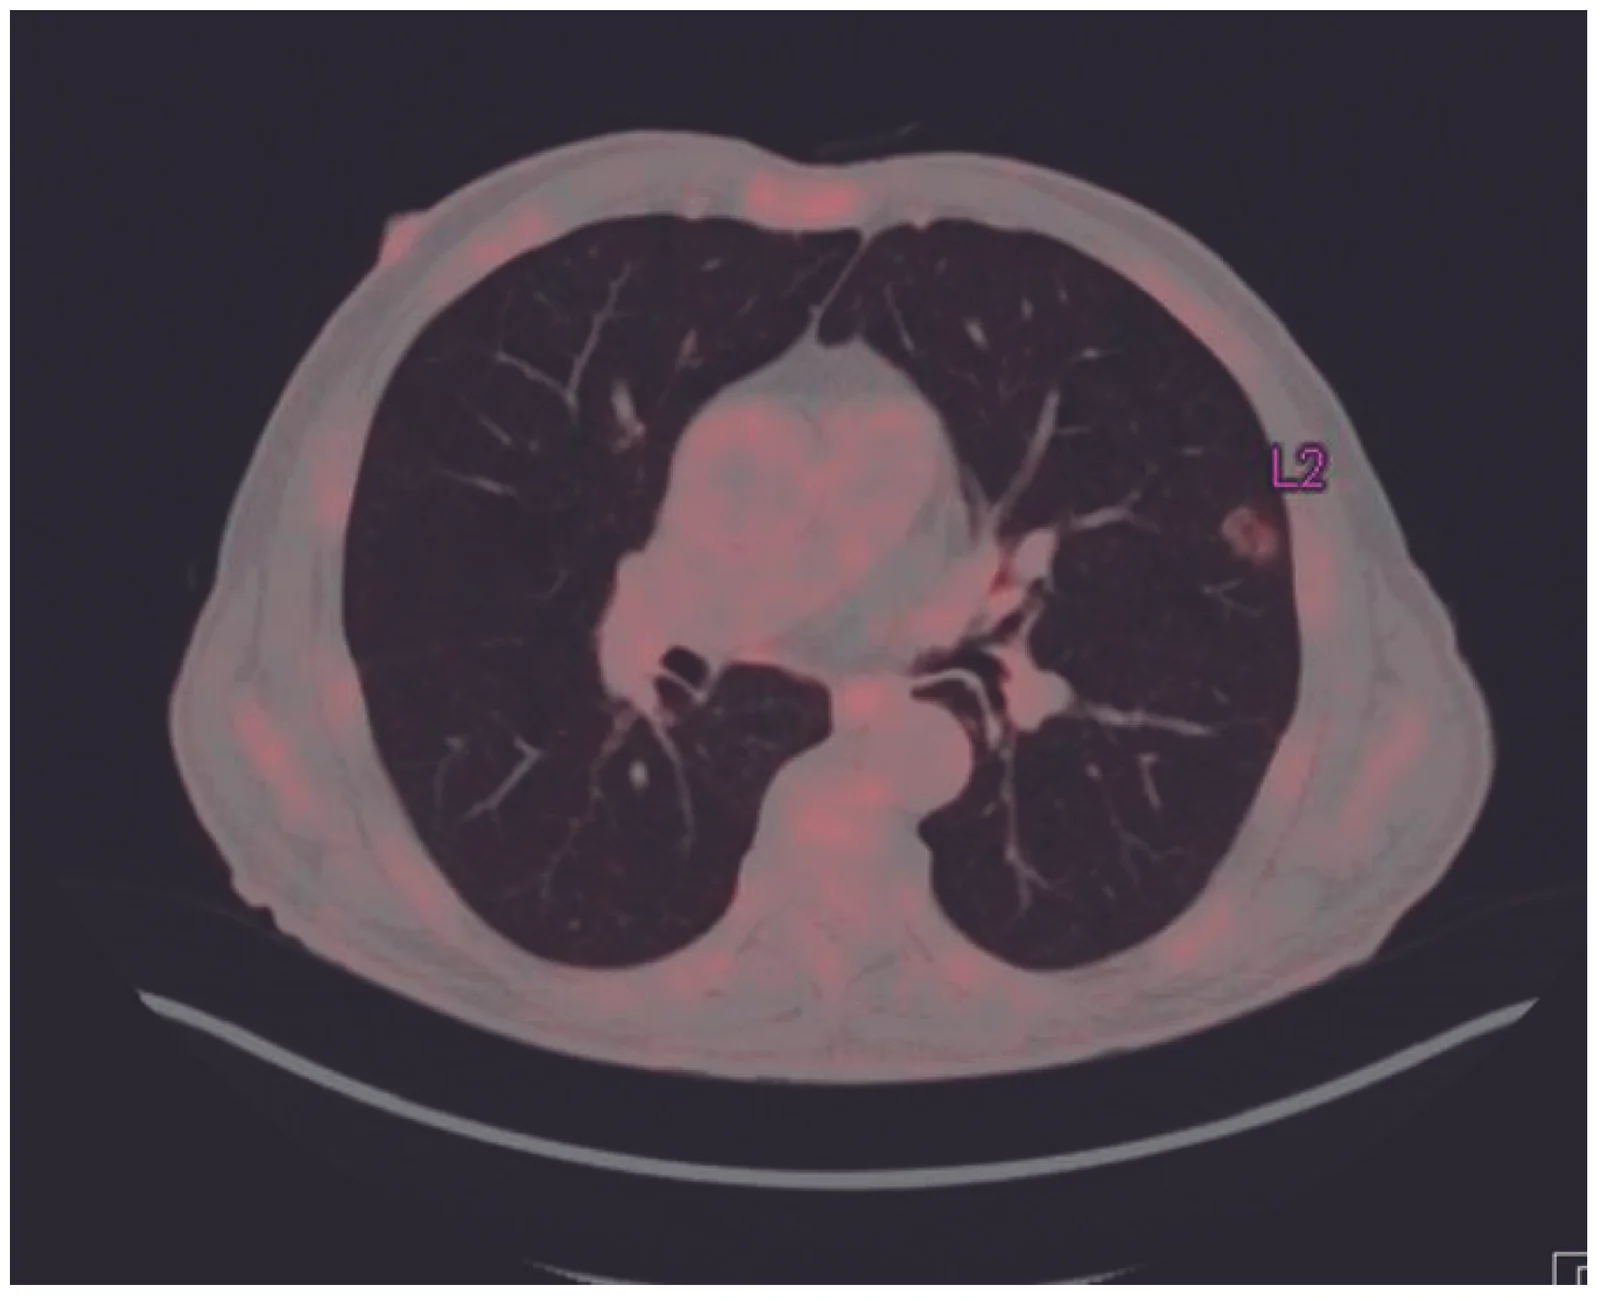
PET/TAC con F18-FDG. Leve crecimiento de un nódulo pulmonar en lóbulo superior izquierdo con patrón metabólico de baja intensidad (L2).
